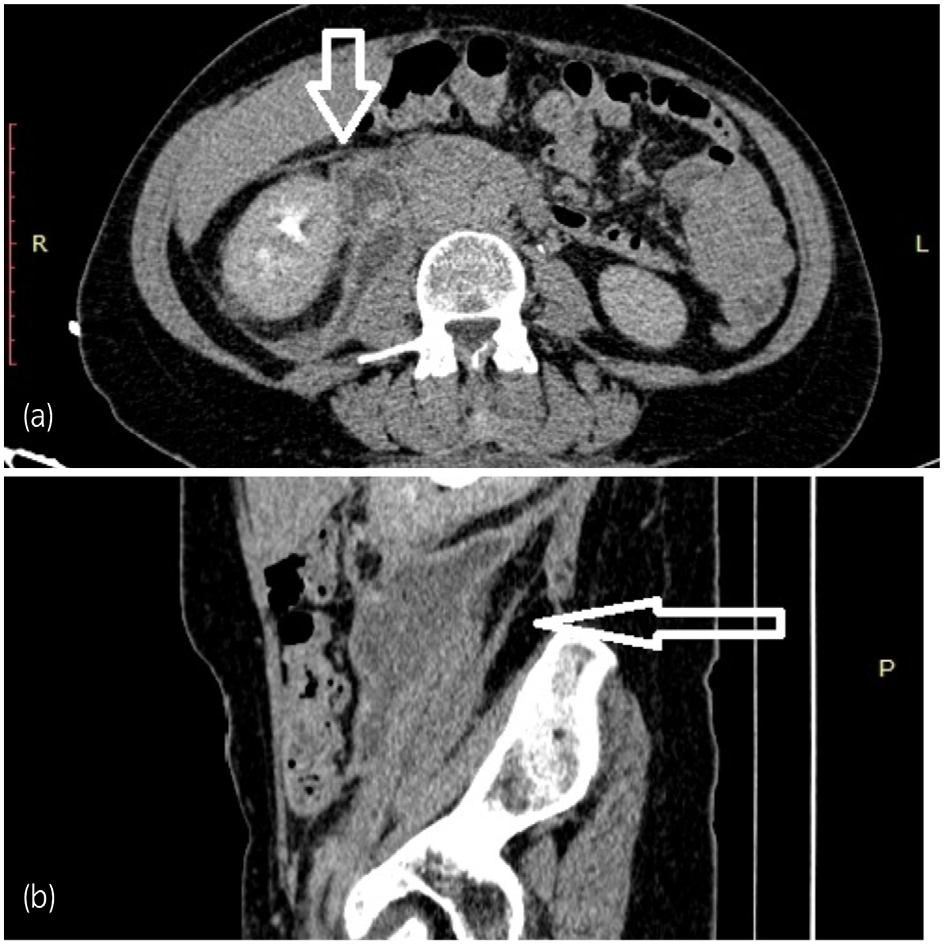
CT urography revealed urinary extravasation from right ureter (a) transversal plane; (b) sagittal plane. Arrow points out the urinoma formation.
